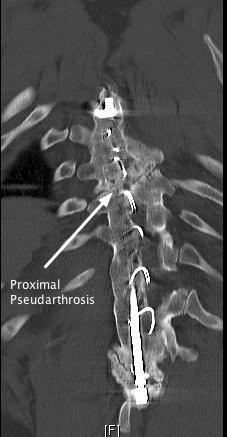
A coronal computed tomography image of the proximal hook and proximal pseudarthrosis. When this figure is compared with Figure
